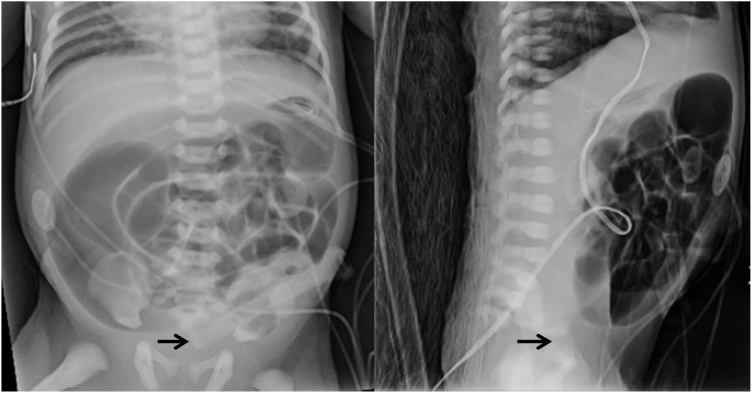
AP and lateral x-ray on day of life 0. Demonstrating passage of oral gastric tube into the gastric fundus, distended loops of bowel, and lack of air passage into the distal rectum (arrows).
